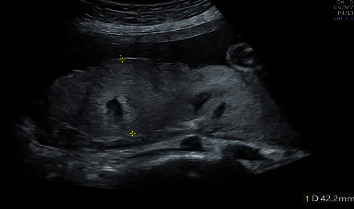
The thickness was 42.2 mm. Placenta by ultrasonography at the gestational age of 25 weeks.
